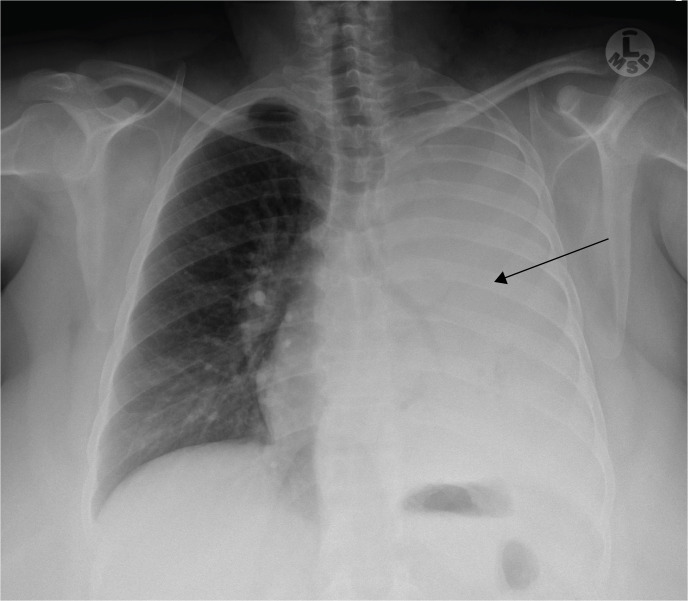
Chest X-ray image showing complete opacification of the left lung.
